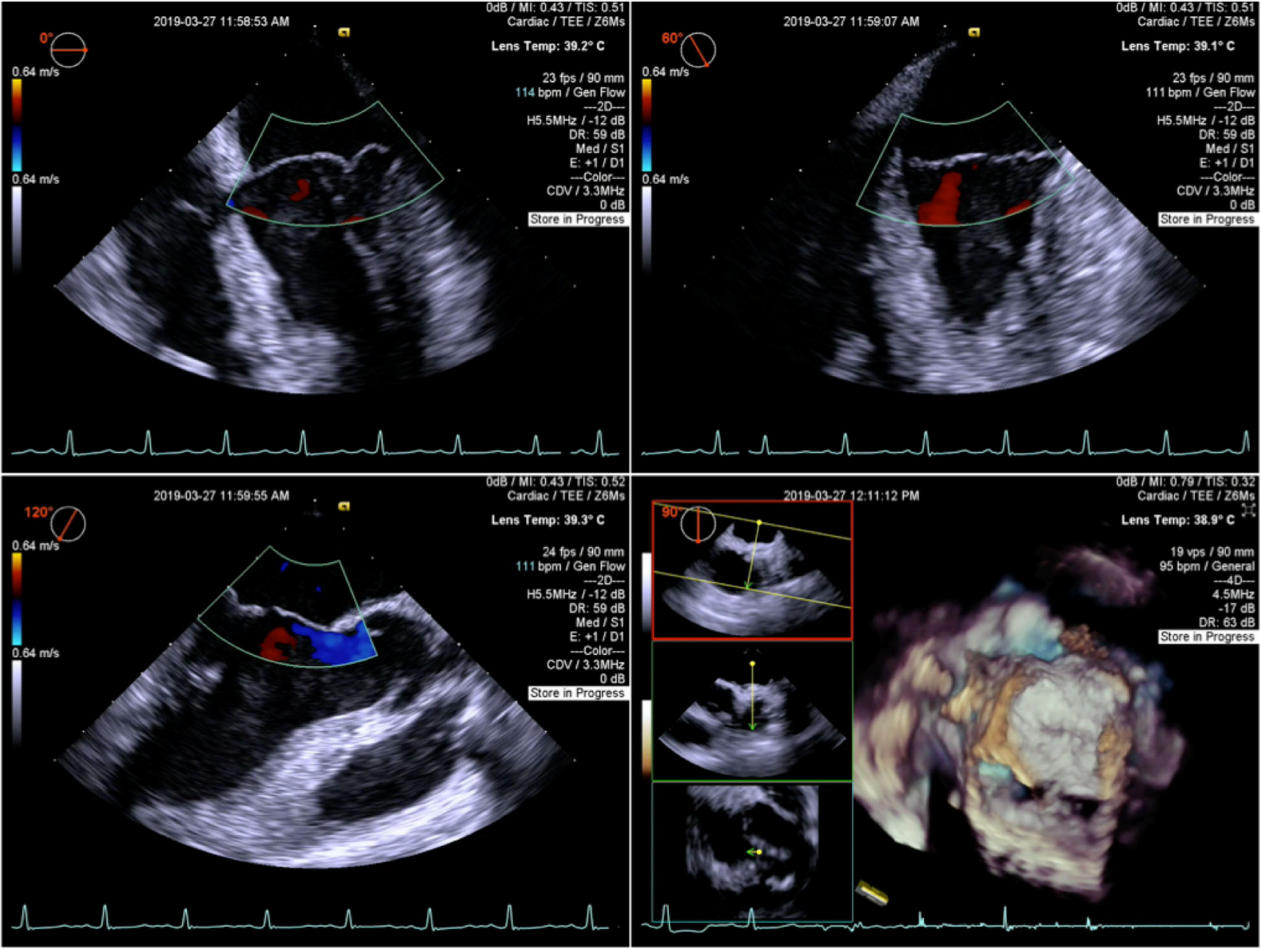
After resection of myxoma, no mitral regurgitation or shunt flow across the atrial septum was revealed by transesophageal echocardiography
